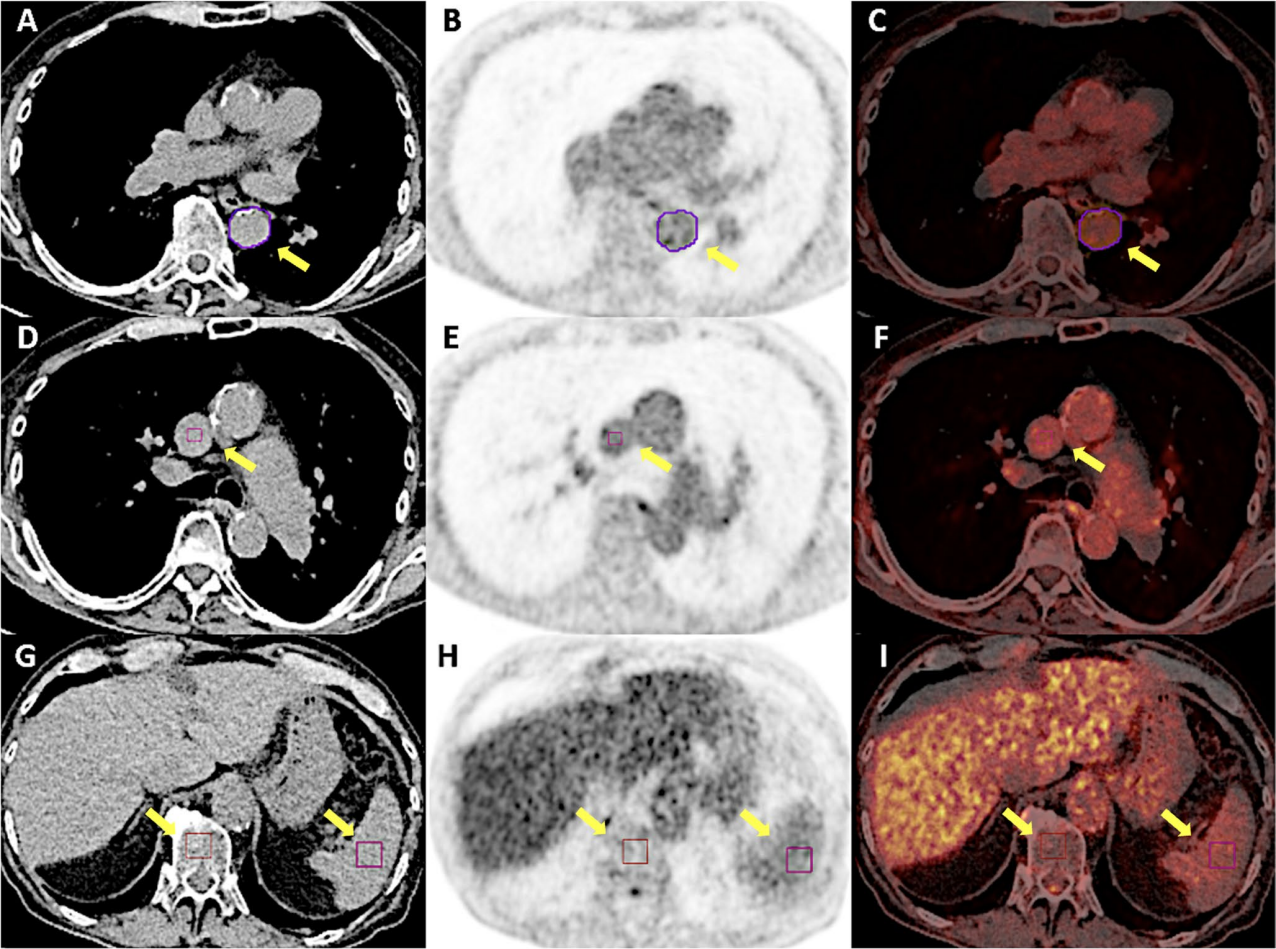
[^18^F]FDG-PET/CT image analysis: An example of image analysis is shown using transaxial CT (A, D, G), PET (B, E, H), and fused [¹⁸F]FDG-PET/CT (C, F, I) images. A circular ROI was manually drawn around the thoracic descending aorta (A–C) on the CT images, and SUV_mean_ and SUV_max_ values were extracted from the corresponding PET images using this ROI. Additionally, cuboidal VOIs were placed in the superior vena cava (D–F), spleen, and bone marrow (G–I) for each patient
